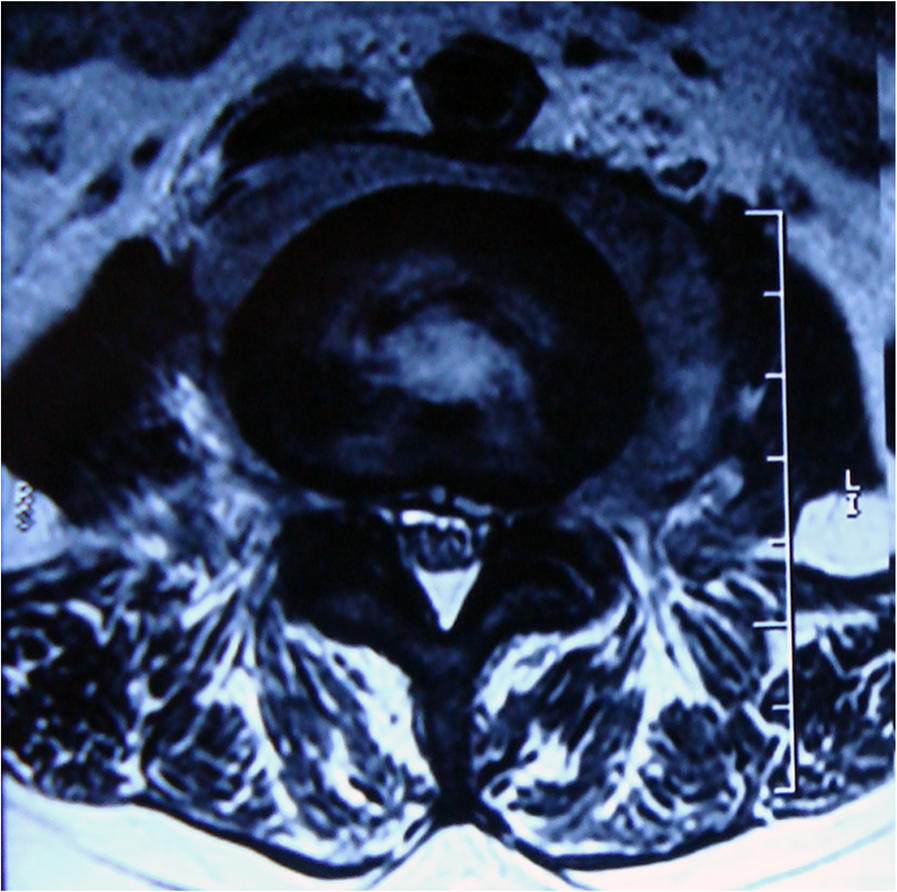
MRI of the lumbar vertebra taken in November 2010. The decreased mass and the alleviated spinal cord compression.
